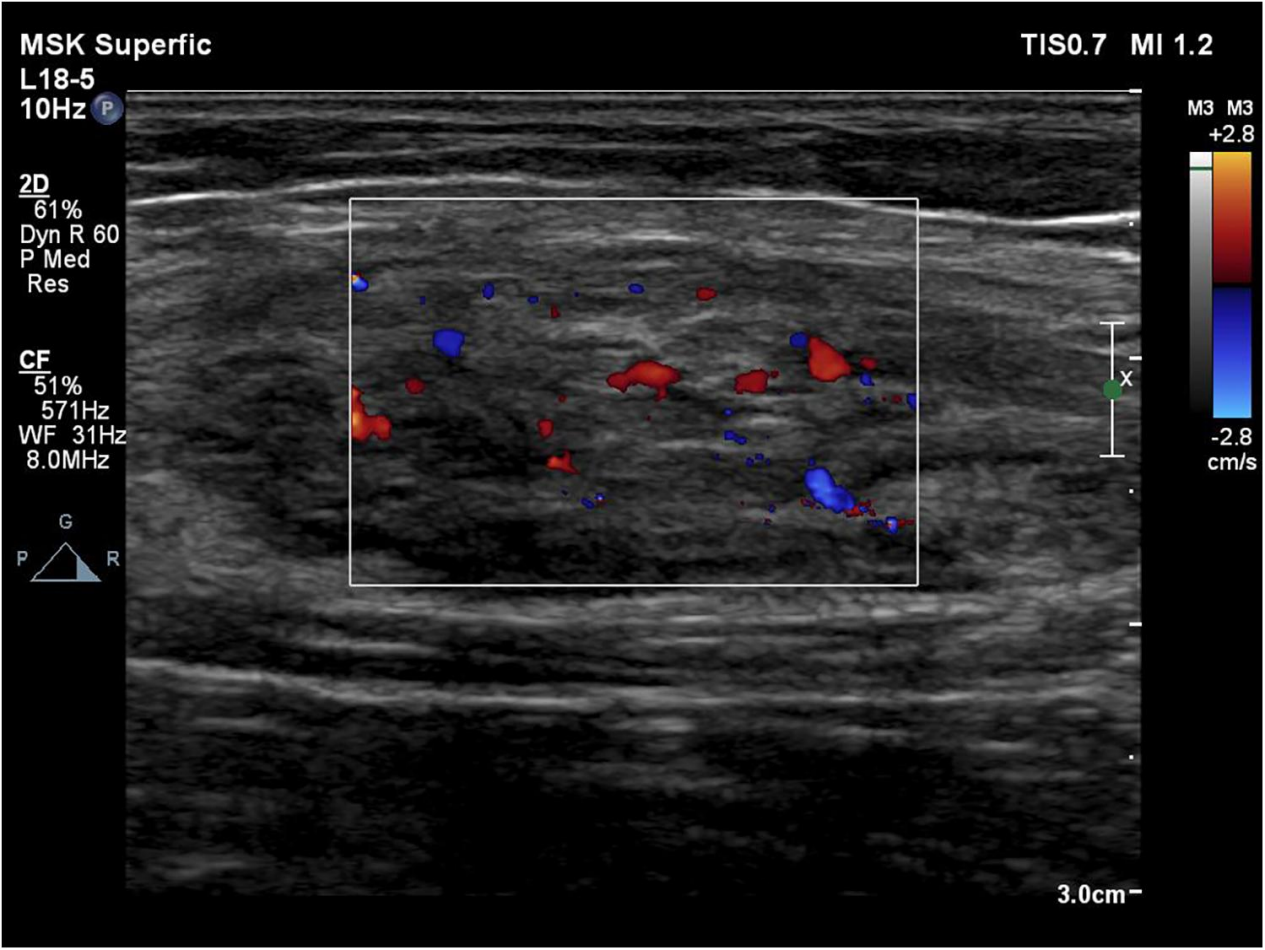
Color Doppler indicates a subcutaneous mass with inhomogeneous echogenicity with dot-like flow signals detected inside.
